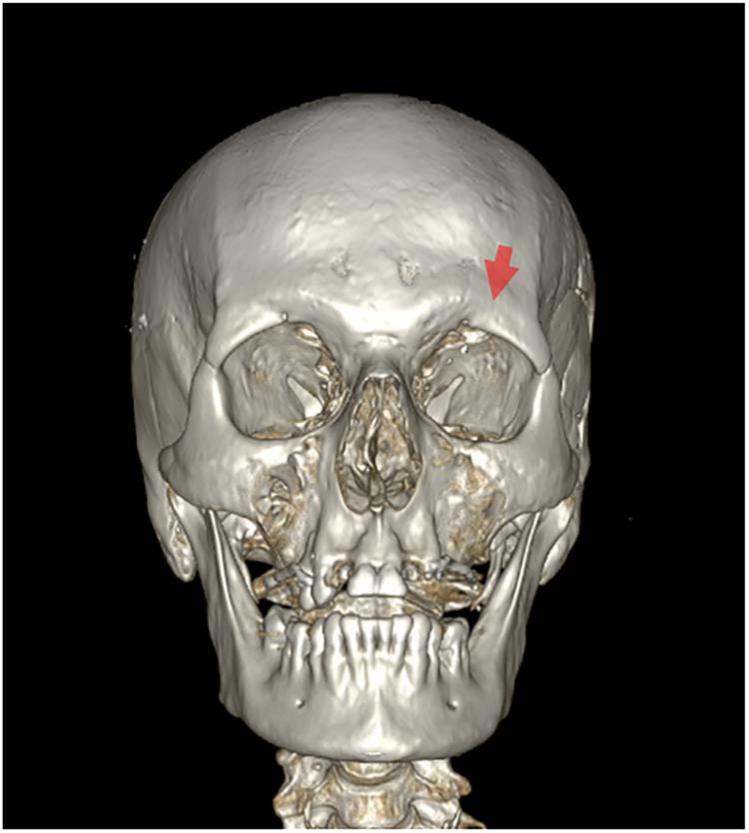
3D reconstruction of the skull (multidetector computed tomography scan before autopsy), showing the front side of the skull before autopsy. No fracture is visible on the left frontal bone, above the left orbit (red arrow).
